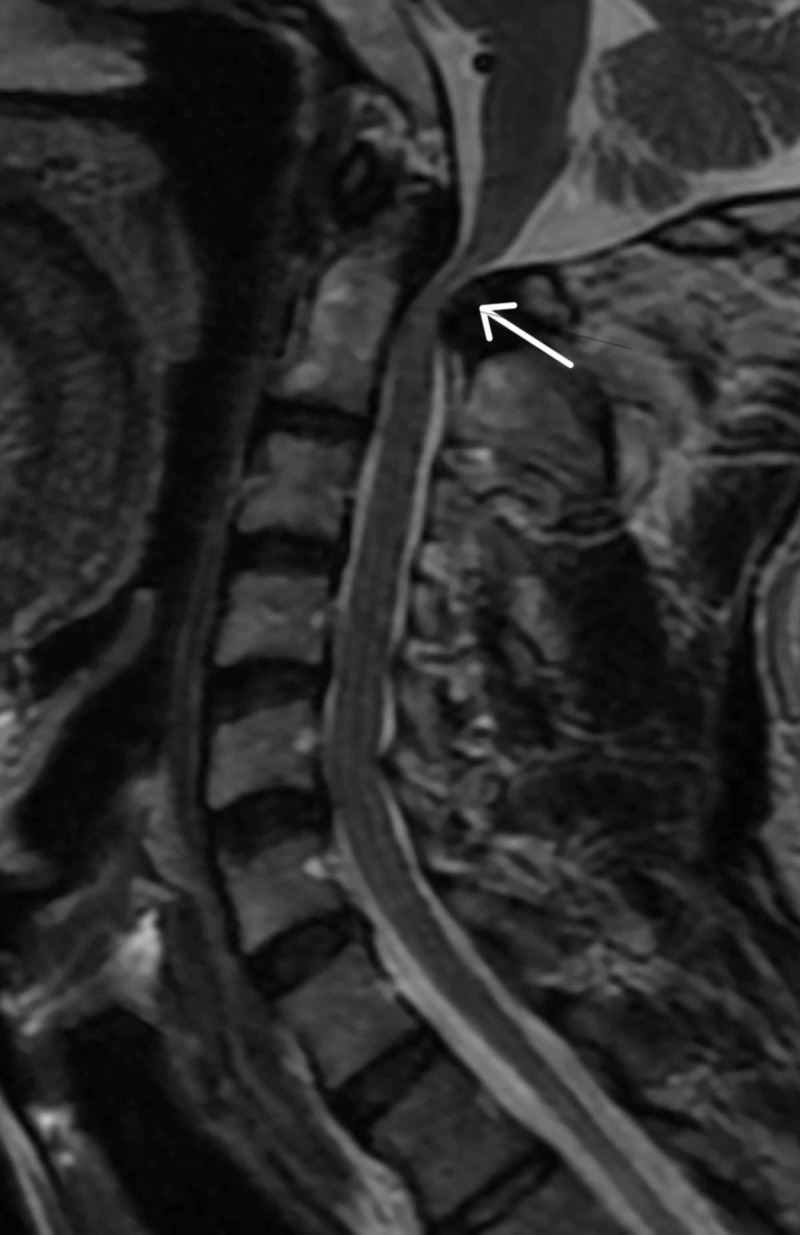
Preoperative T2-weighted MRI of the cervical spine with severe cervical stenosis and cervical myelopathy Preoperative MRI showing severe canal stenosis at C1 with evidence of myelomalacia.
